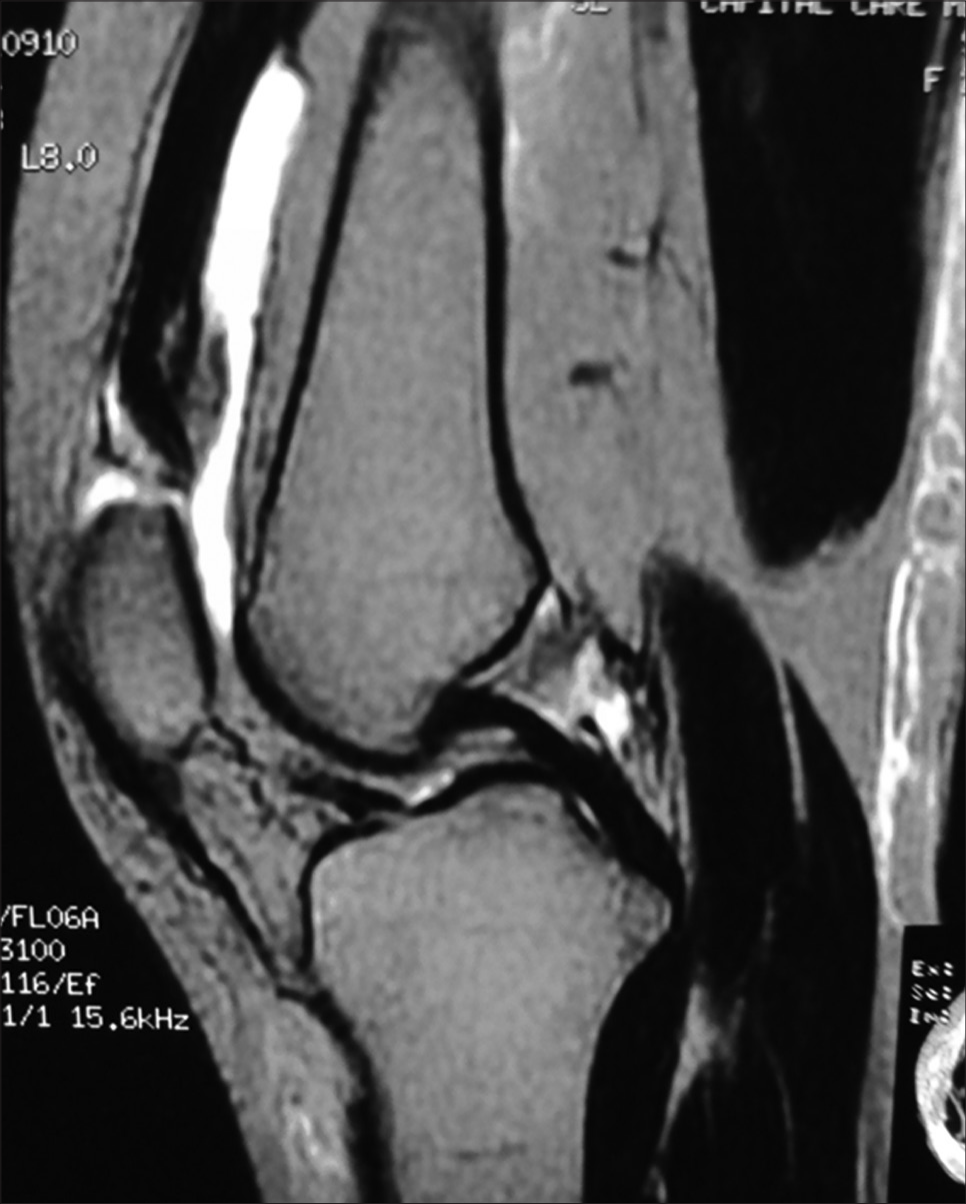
T2-weighted sagittal MR image of right knee showing discontinuity between lower end of hypointense signal intensity quadriceps tendon and upper pole of patella with intervening hyperintense signal intensity; joint effusion is seen
